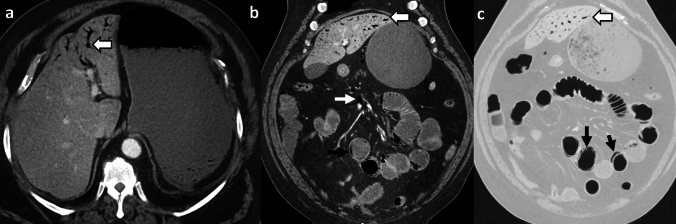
Life-threatening pneumatosis intestinalis. A 47-year-old with sudden-onset abdominal pain. CT images show portal venous gas (white block arrows, a–c) in peripheral liver, gas in the superior mesenteric vein (white arrow, b), and dilated small bowel with pneumatosis intestinalis (black arrows b, c). Serum lactate and WBC counts were elevated, and the patient was taken for laparotomy. Intraoperatively, there was dilation of the jejunum with evidence of ischemia
